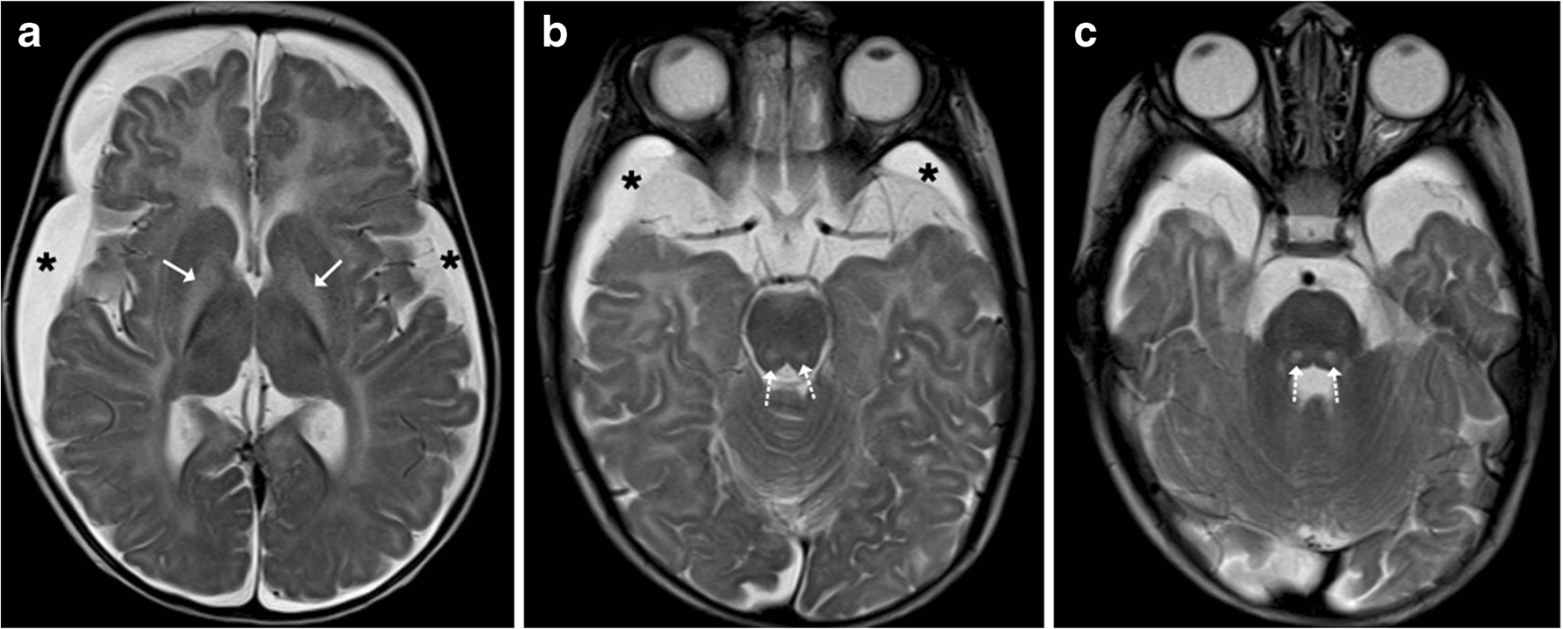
An 8-month-old child with glutaric aciduria presenting with altered sensorium: Axial T2-weighted image shows hyperintensity involving the globus pallidi (*arrow*) and bilateral subdural hygromas (*asterisk*). Prominence of the CSF spaces is noted anterior to the temporal lobe. Increased signal intensity is noted in the pontine tegmentum (*dotted arrow*)
